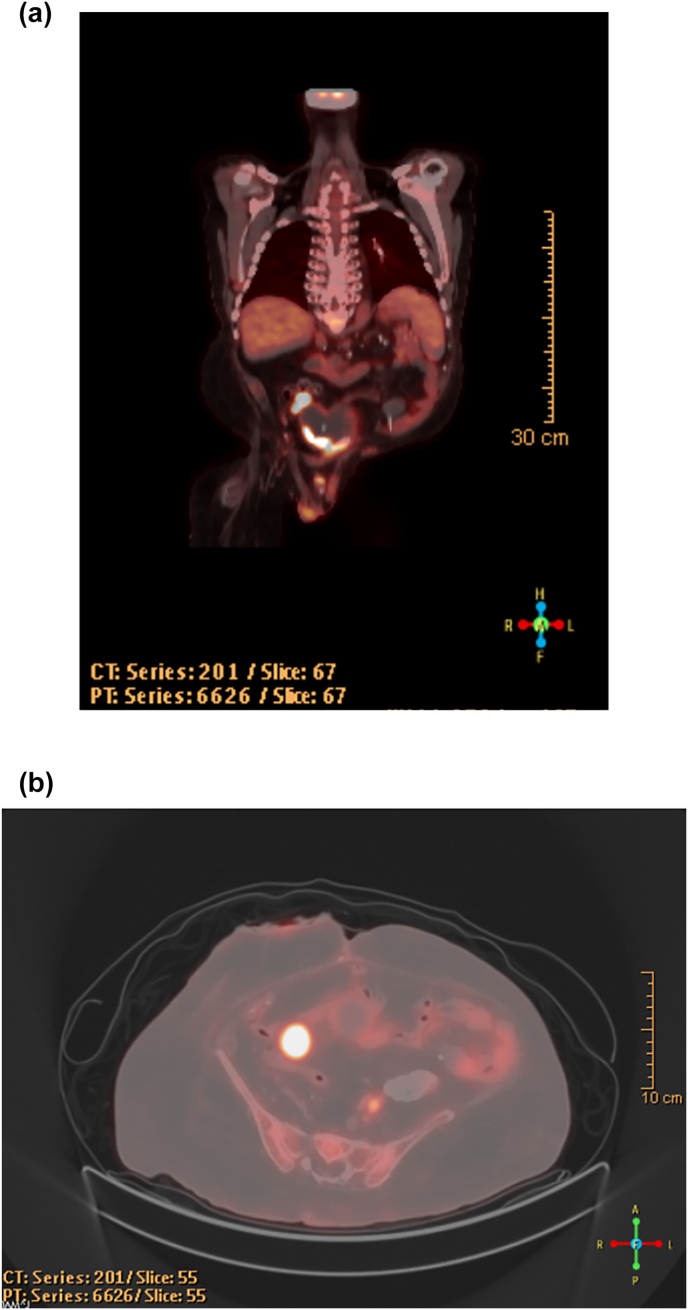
PET CT Images from 2/12/25 showing suspicious left-sided presacral lymph node. Coronal(a) and transverse views(b).
